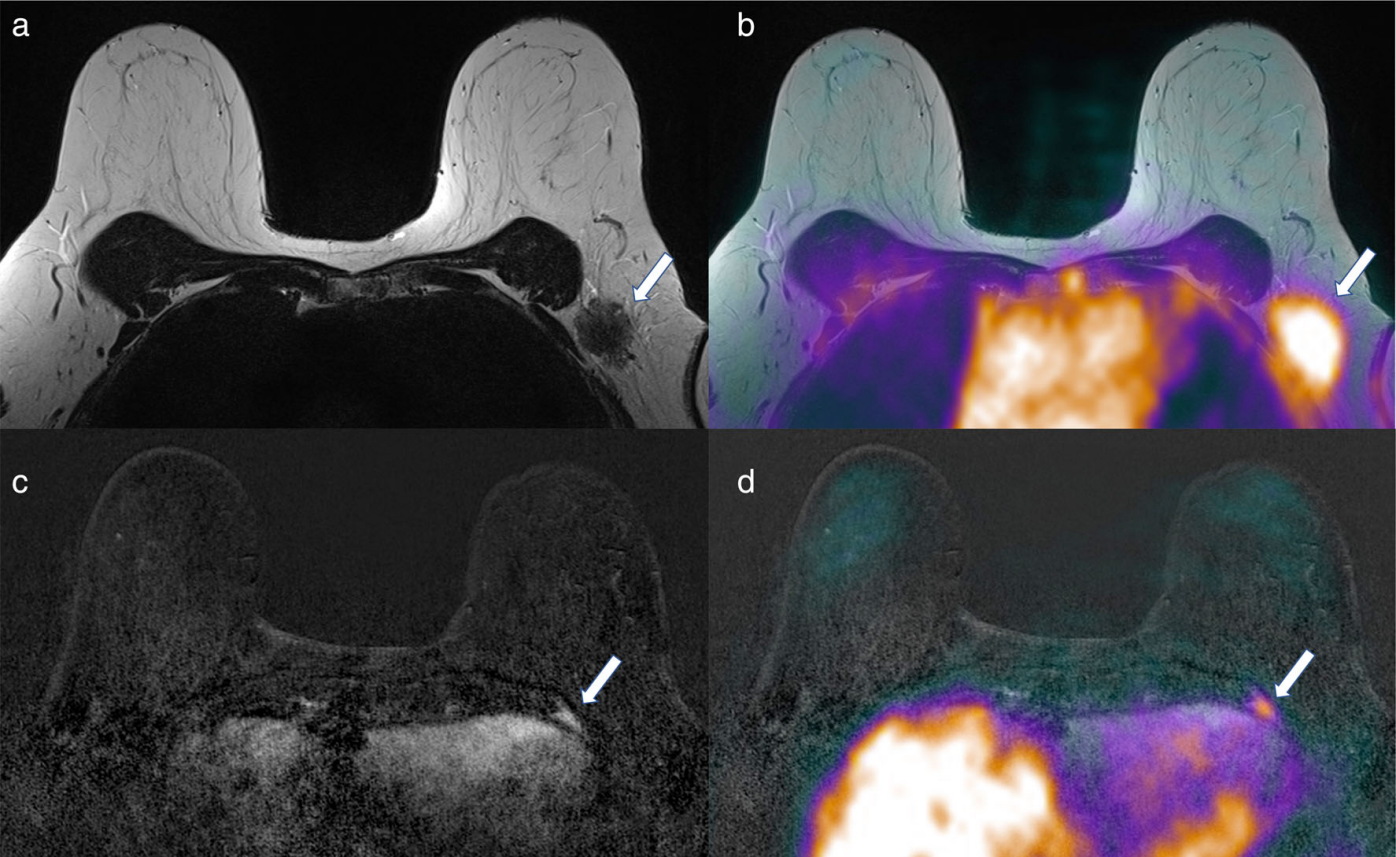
Axial (a) T2‐weighted, (b) fused T2‐weighted and PET, (c) subtracted DCE‐MRI, and (d) fused DCE‐MRI and PET imaging. A heterogeneous axillary metastasis (arrows in a and b) and a rib bone metastasis (arrows in c and d) are detectable in a 66‐year‐old patient with invasive ductal breast cancer (G3, ER/PgR−, HER2+) of the left breast (same patient as Fig. 3).
